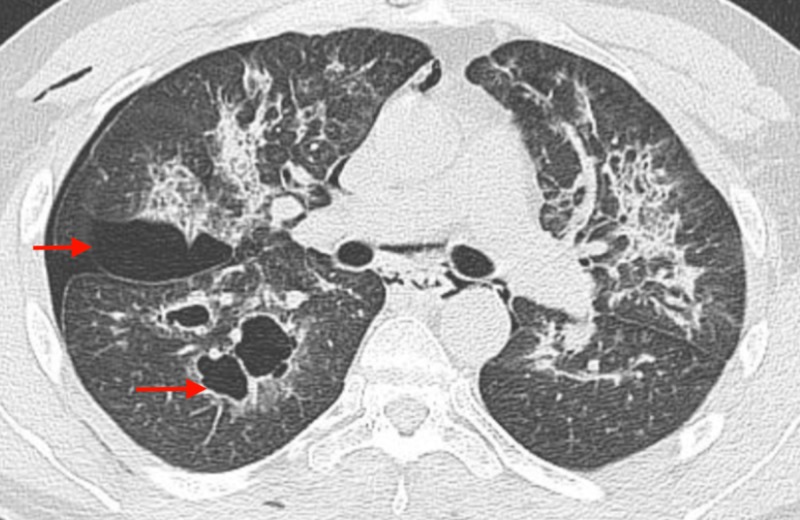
CT scan of the chest showing blebs on the right side (red arrows).
